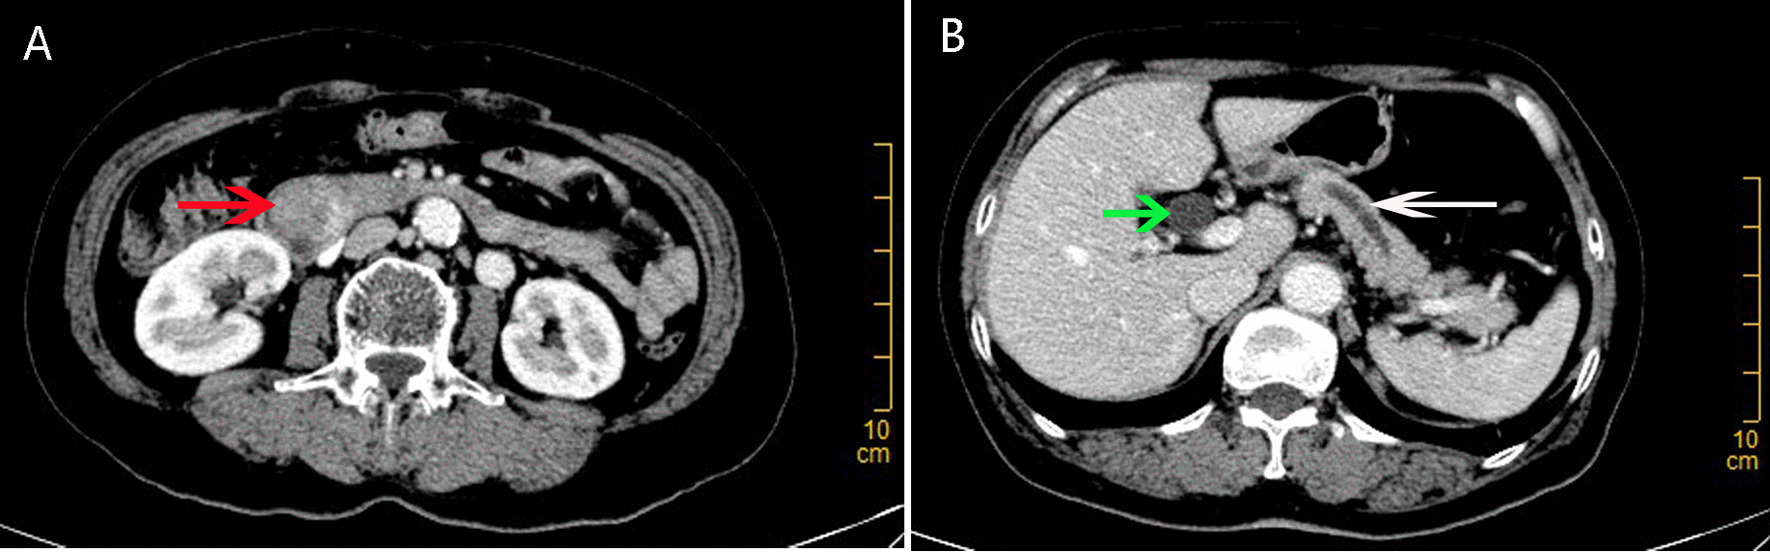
Abdominal contrast-enhanced CT revealed a hype-vascular tumor and dilation of the extrahepatic bile duct and pancreatic duct. A Axial CT image in the arterial stage showed a hype-vascular tumor (red arrow) located in the descending part of duodenum; B Axial CT image in the arterial stage showed dilation of the extrahepatic bile duct (green arrow) and pancreatic duct (white arrow)
